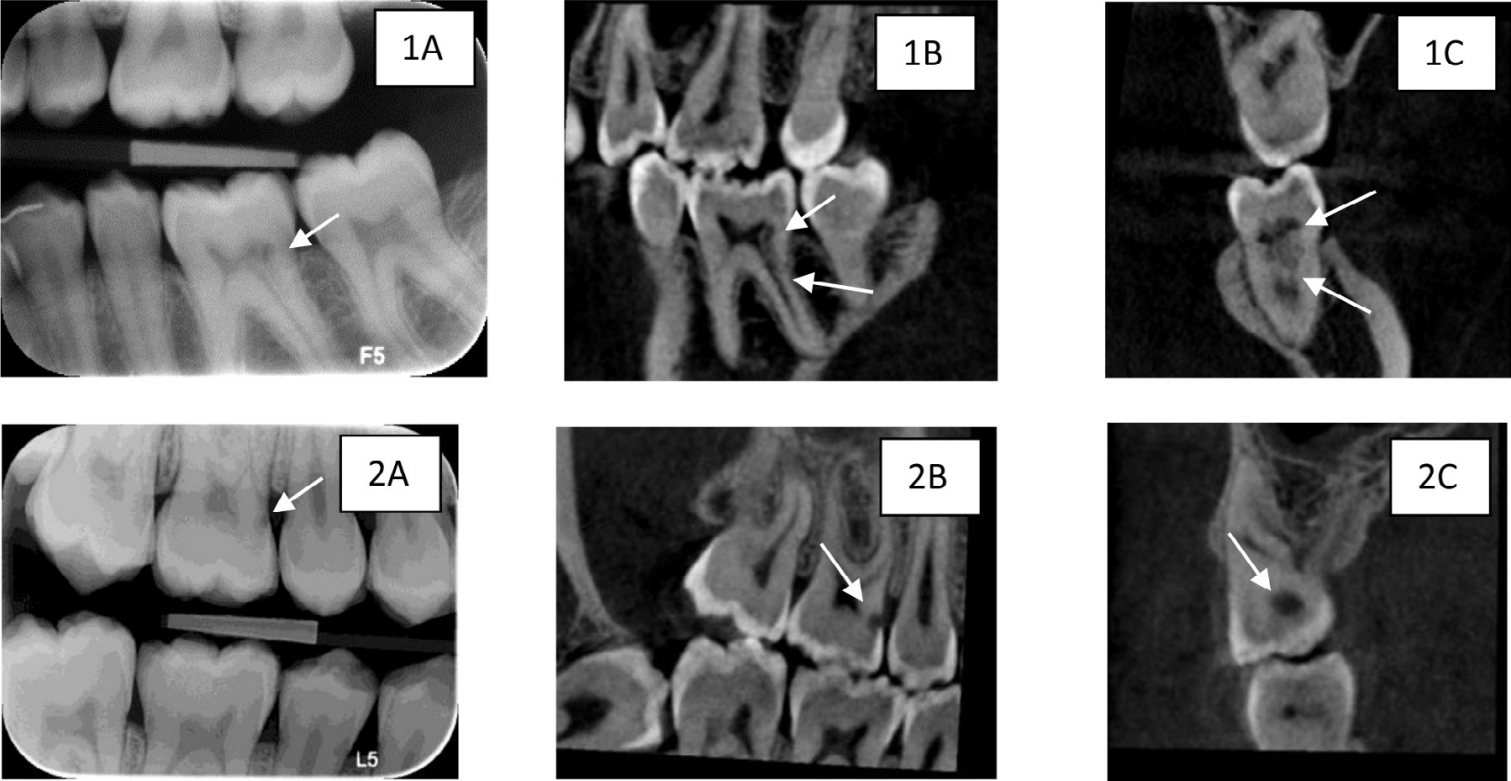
Example of ECR that changes severity assessment from BW to CBCT. Case 1: a lower left side molar where severity assessment changed from class 3 in BW to class 4 in CBCT. 1A: BW; 1B: CBCT section in the sagittal plane; 1C: CBCT section in the coronal plane. Case 2: an upper right side molar where severity assessment changed from class 1 in BW to class 2 in CBCT. 2A: BW; 2B: CBCT section in the sagittal plane; 2C: CBCT section in the coronal plane. BW, bitewing; CBCT, cone beam CT; ECR, external cervical resorption.
